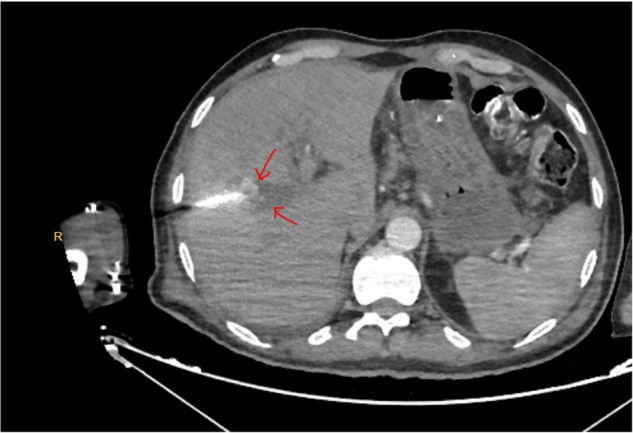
Contrast-enhanced CT angiography demonstrating active contrast extravasation from a pseudoaneurysm.
